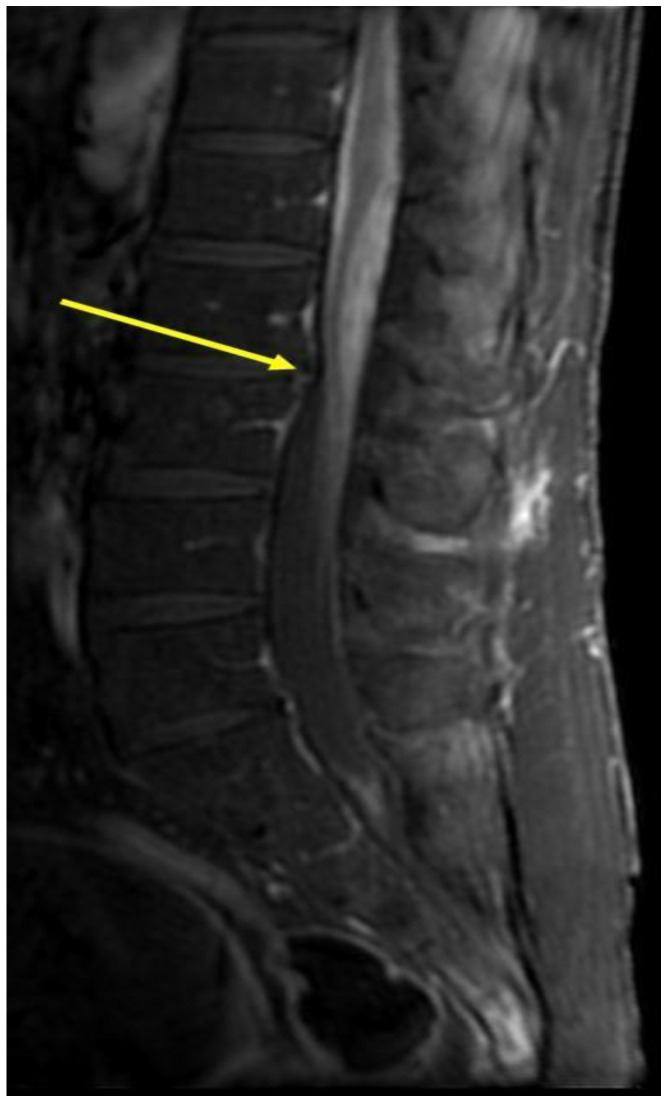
MRI scans with contrast of the second patient before the anti-TNF-α treatment. Spinal MRI of the second patient. Sagittal post-gadolinium T1 MRI of the lumbar spine demonstrating nodular leptomeningeal enhancement about the cauda equina (arrowhead).
